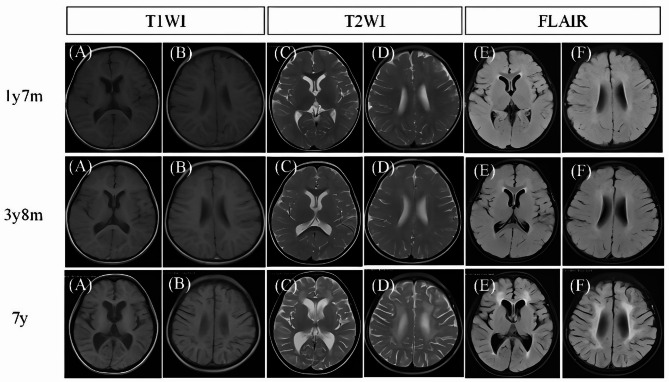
Brain magnetic resonance imaging (MRI) of the proband. At the age of 1 year and 7 months, no obvious abnormalities were detected. Brain MRI at 3 years and 8 months indicated decreased white matter volume and hypomyelination. At the age of 7 years, (A, B) Deepened sulci in the bilateral cerebral hemisphere. (C, D) T2WI showed significantly decreased white matter of bilateral cerebral hemispheres. (E, F) Patchy FLAIR high signals around the bilateral ventricles
